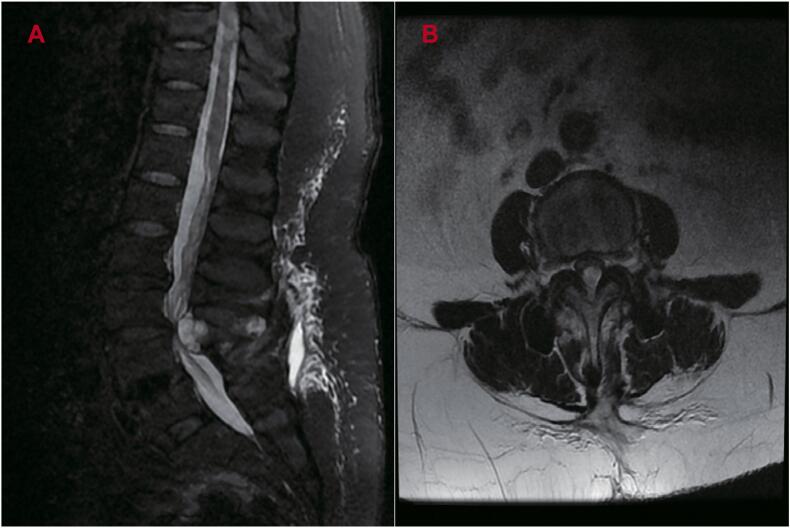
A-B. Sagittal (A) and axial (B) MR images showed lateral recess stenosis at the L3/4 segments secondary to synovial cysts.
